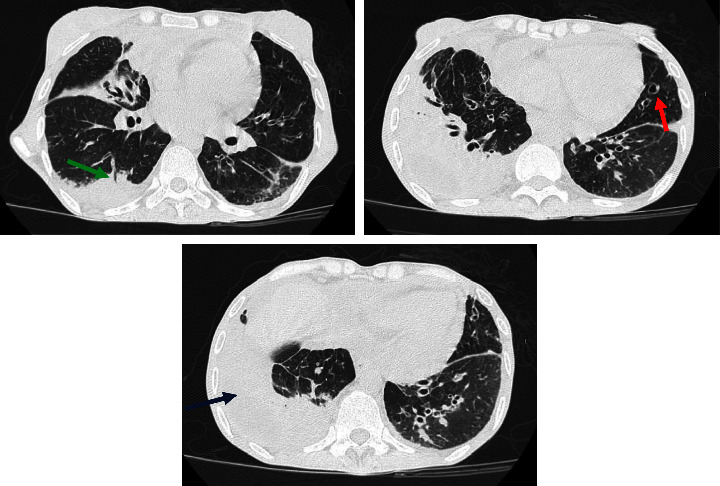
Three different sections of lung CT scan of the patient at admission time showing pleural effusion (blue arrow), consolidation (green arrow), and bronchiectasis (red arrow).
